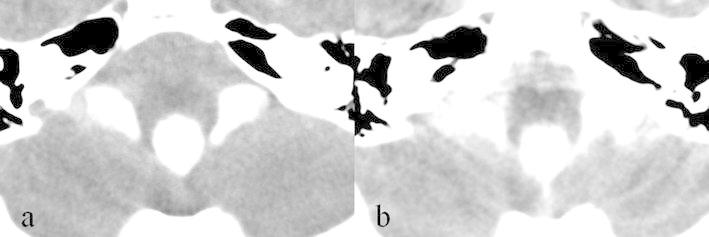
Computed tomography images after the injection of contrast medium through the ventricular catheter (a 1 h after injection, b 3 h after injection). Both images show delayed diffusion of contrast medium to the pre-pontine cistern
